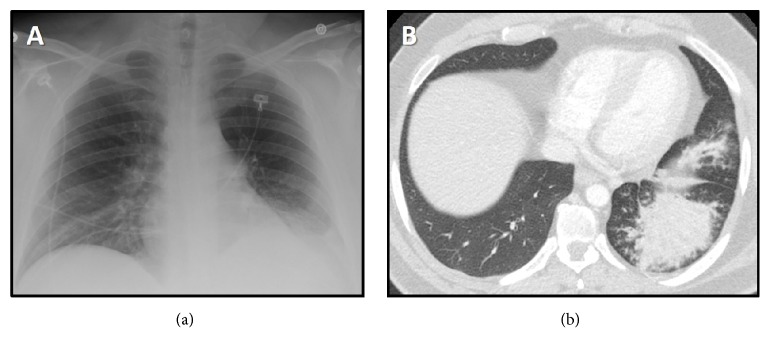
Chest radiograph (a) and computerized tomography (b) demonstrate left basilar lung opacification.
